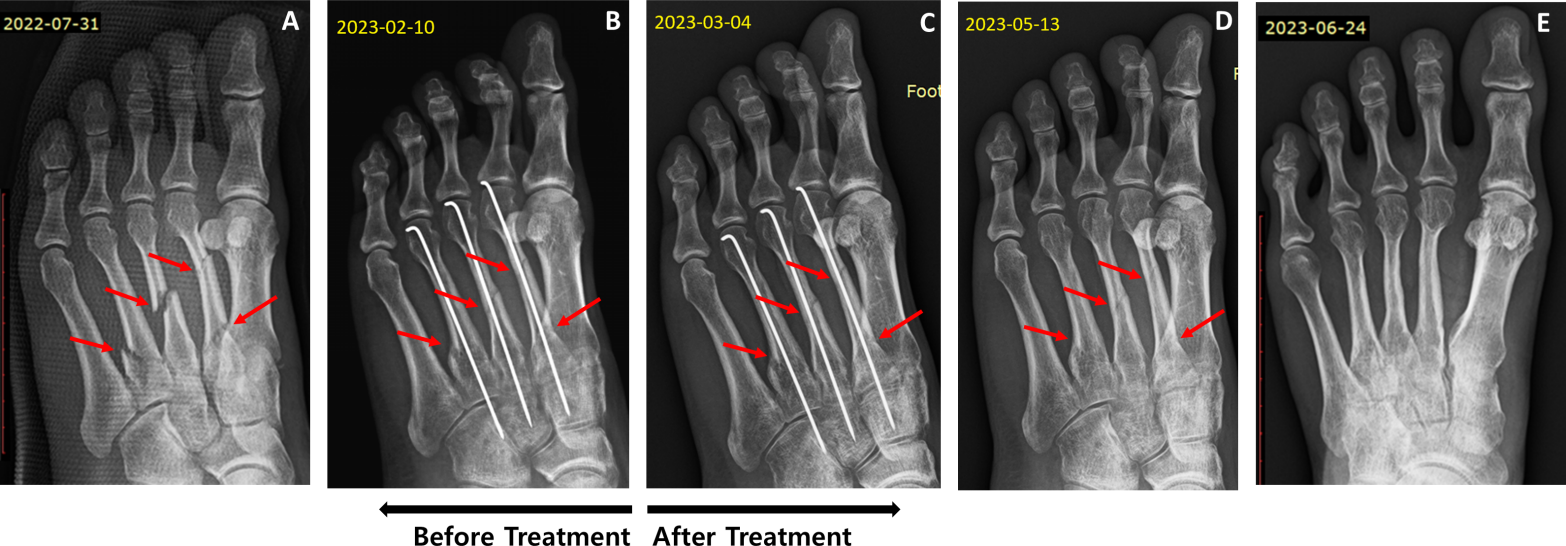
Radiographic images of the left foot of Case 2, a 30-year-old woman with multiple metatarsal fractures in the left foot. (A) Fracture condition before surgery (July 31, 2022); (B) Fracture status 6 months after surgery (February 10, 2023); (C) Fracture condition 1 month after JGT administration (March 4, 2023); (D) Fracture condition 3 months after JGT administration (May 13, 2023); (E) Bridging callus density similar to the bony cortex (June 24, 2023).
